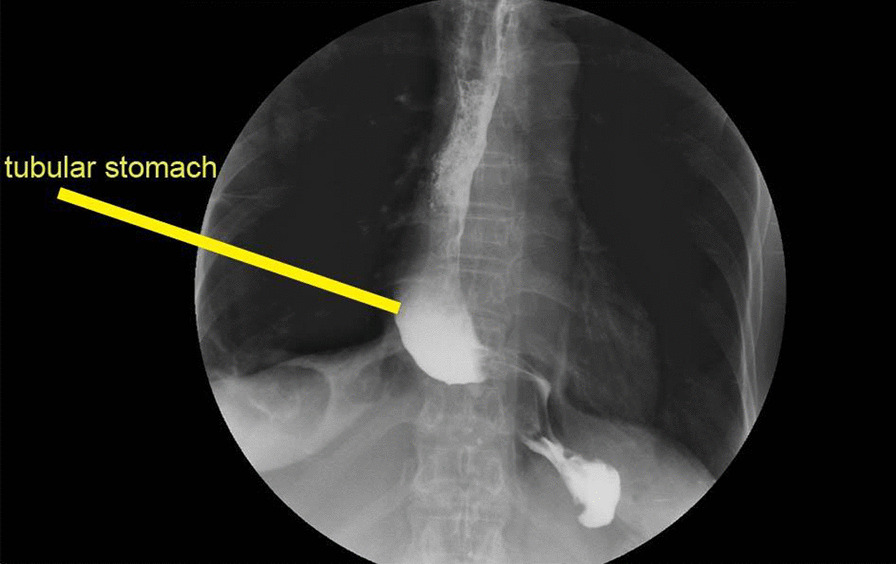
X-ray findings of upper gastrointestinal angiography on the 7th postoperative day
